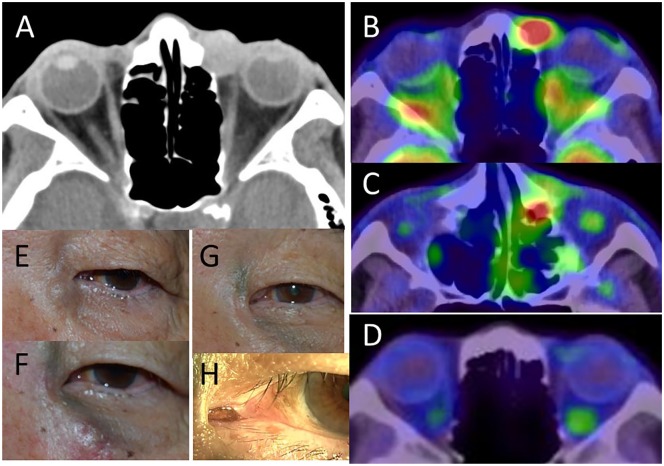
Lacrimal sac mass (E) on the left side on computed tomographic scan (A) at
